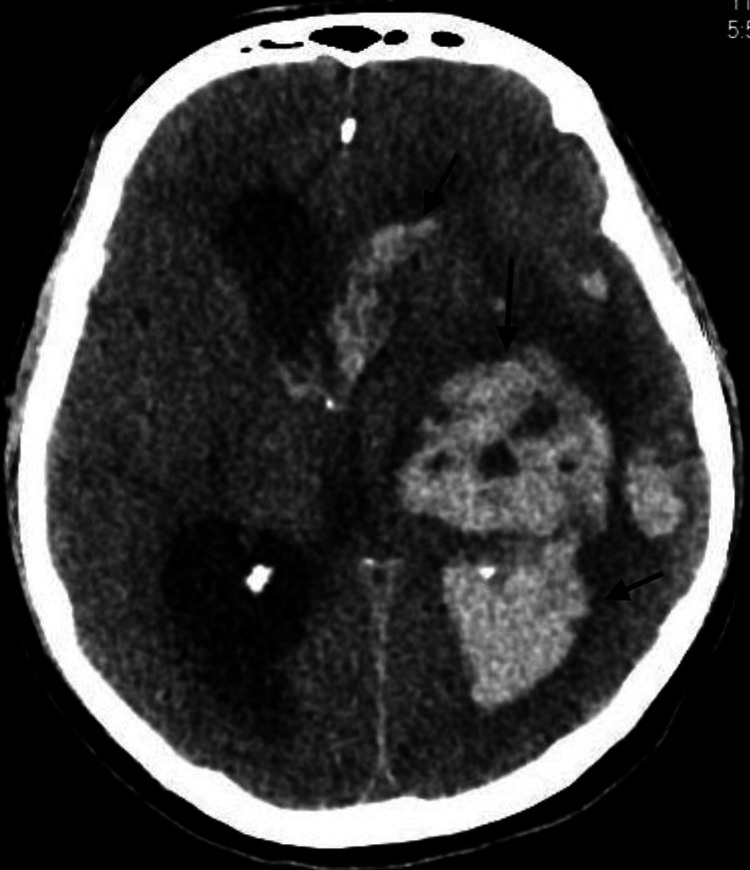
Hemorrhagic transformation of abscesses on CT imaging without contrast, following a drastic change in the neurological exam.
